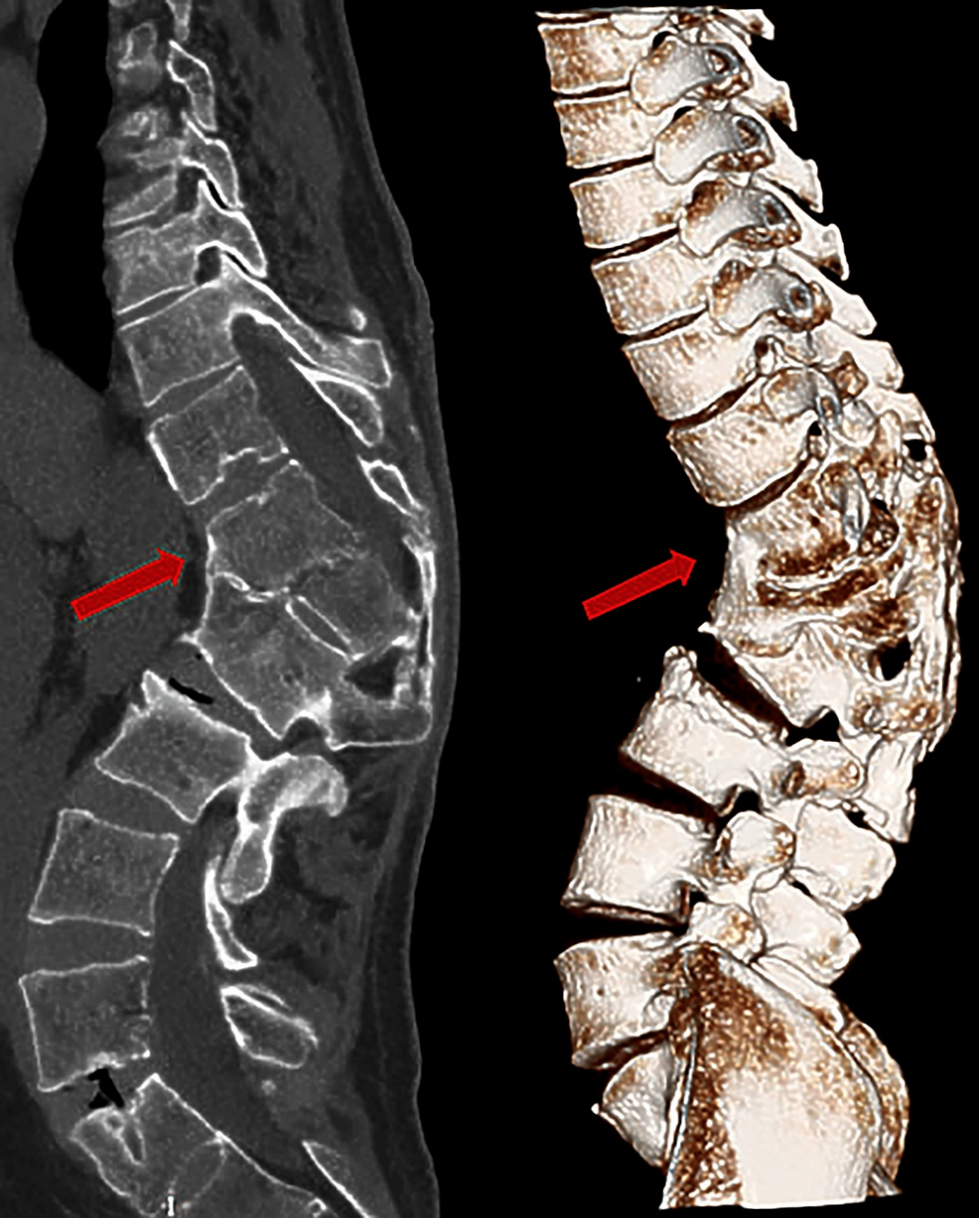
The patient's spine MRI (left) and spine CT three-dimensional reconstruction (right) showed severe scoliosis (arrows).
